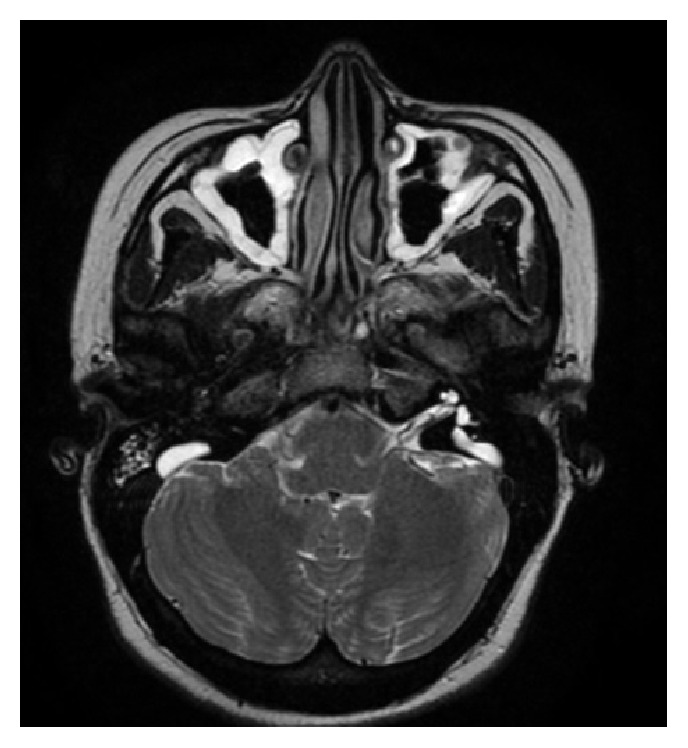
Magnetic resonance imaging of the inner ear showing the bilateral large vestibular aqueduct was demonstrated.
